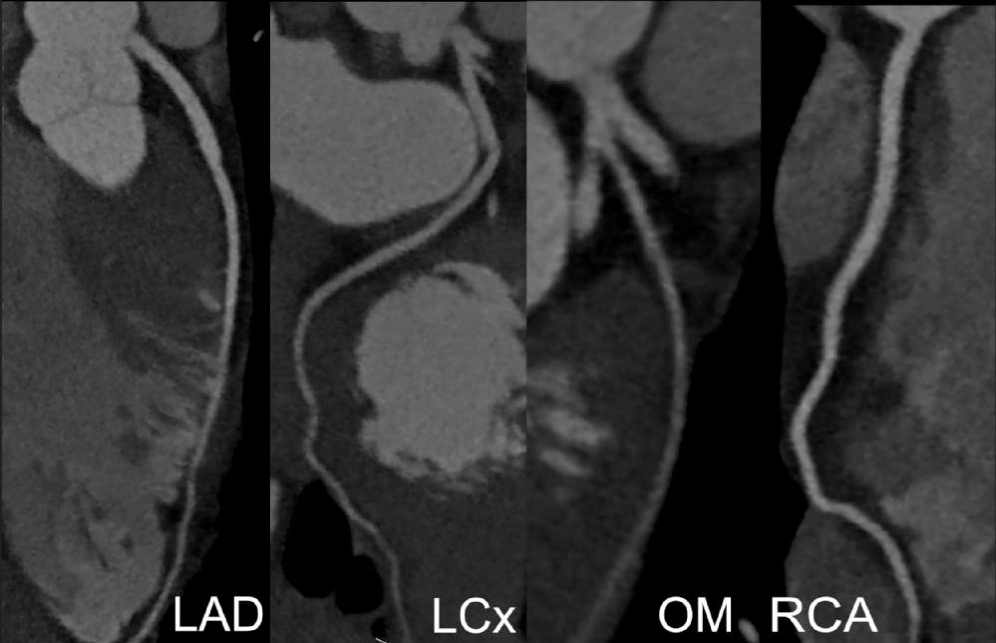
Coronary computed tomography angiography. The coronary computed tomography angiography showed no obvious signs of arterial disease or other abnormalities. LAD, left anterior descending; LCx, left circumflex; OM, obtuse marginal; RCA, right coronary artery.
